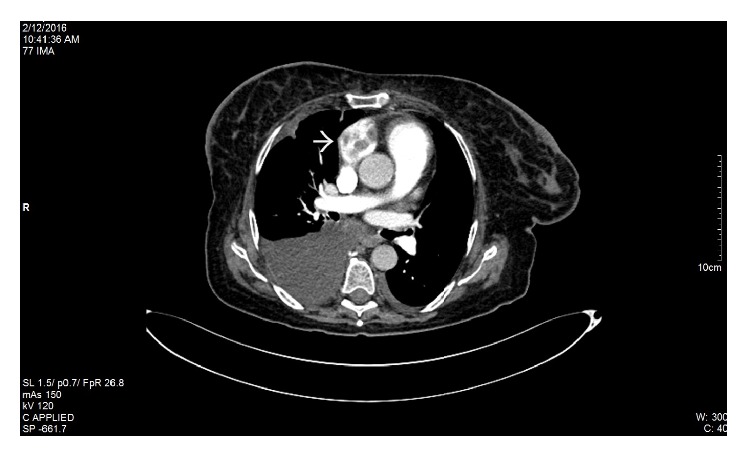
Contrast-enhanced CT scan. The white arrow shows multiple thrombi in the left atrium.
